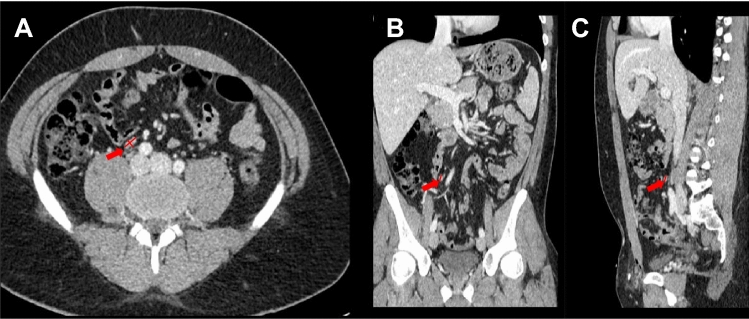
A 27-year-old patient with unclear retroperitoneal lymph nodes at CT staging (right-sided nonseminomatous germ cell tumors (NSGCT) (yolk sack tumor), pT1, L0, V0, R0 A. Unclear retroperitoneal lymph node at the critical landing zone with an LAD of 12 mm and SAD of 5 mm in the axial plane. B The same lymph node with an LAD of 9 mm in the coronal plane and C with an LAD of 9 mm in the sagittal plane. This is an example of an interpretation issue in clinical staging, as this patient could be classified as cN0 or cN1, resulting in a decision between surveillance and further therapy. This patient did not receive any further treatment and followed up imaging showed constant lymph nodes, classifying as non-metastatic cN0. CT images were kindly provided by Dr. Waldeck, Department of Radiology, Federal Armed Services Hospital Koblenz, Germany
